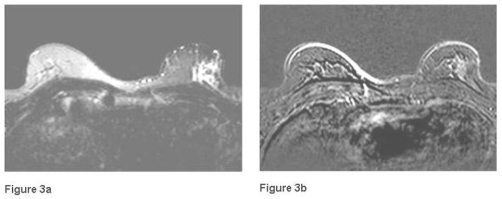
Axial T2-weighted breast MRI (a) et T1-weighted MRI with injection of gadolinium (b) showing a retromamelonnar speculated left lesion measuring 24 mm with a central marked enhancement.
